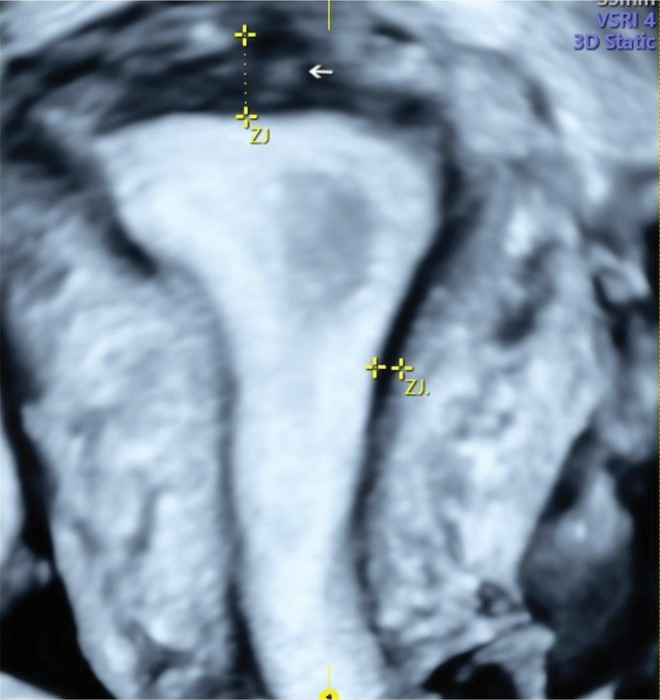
Three-dimensional ultrasound imaging of a uterus in the coronal plane showing focal adenomyosis of the inner myometrium: junctional zone interrupted with one hyperechogenic island (arrows).
